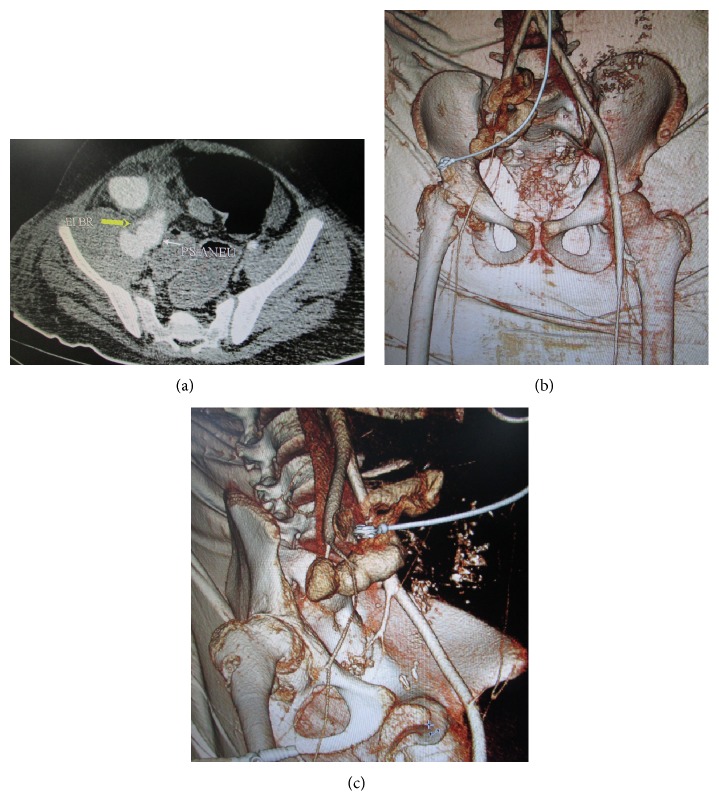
(a) Axial computerised tomography (CT) angiography image showing a branch of external iliac artery (EI BR) feeding the pseudoaneurysm (PS ANEU). (b) and (c) 3D reconstruction CT angiography images showing branch of the external iliac artery feeding the large bowel loops.
